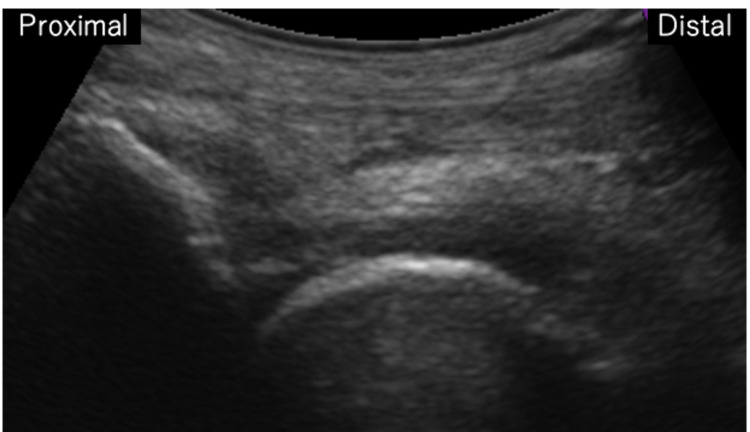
Two-year postoperative follow-up ultrasonography shows the mass in front of the hip joint is disappeared.
